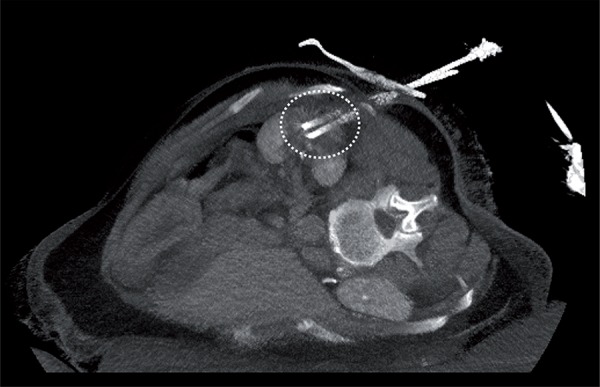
Interventional CT-scan. Cryoablation with two cryoprobes within target lesion. Dotted circle: edge of ice-ball.
